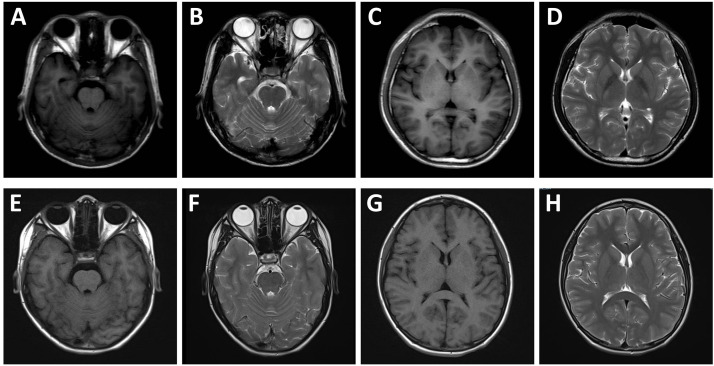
Cranial magnetic resonance imaging (MRI) findings of the patient
